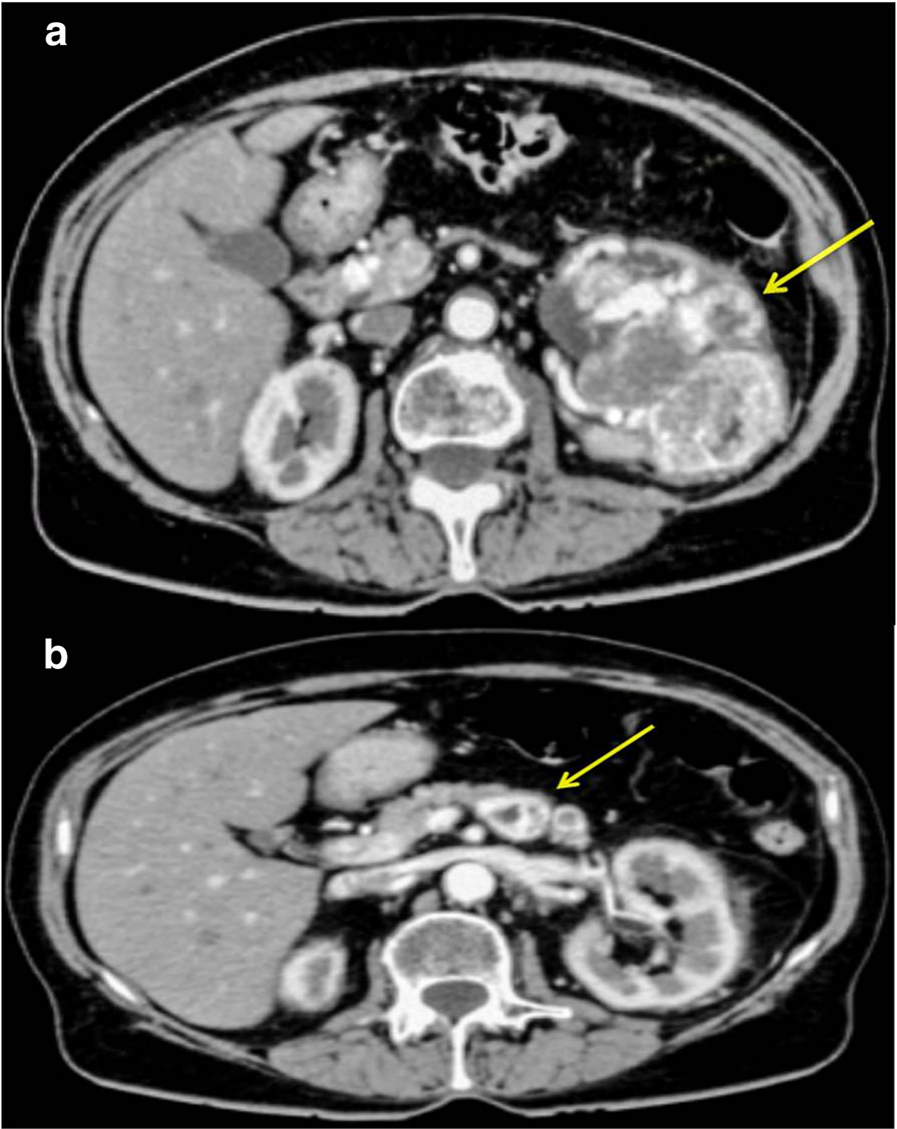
Pre-operative Computed Tomography (CT) scan: yellow arrows indicate the left renal mass (a) and its pancreatic metastasis (b)
